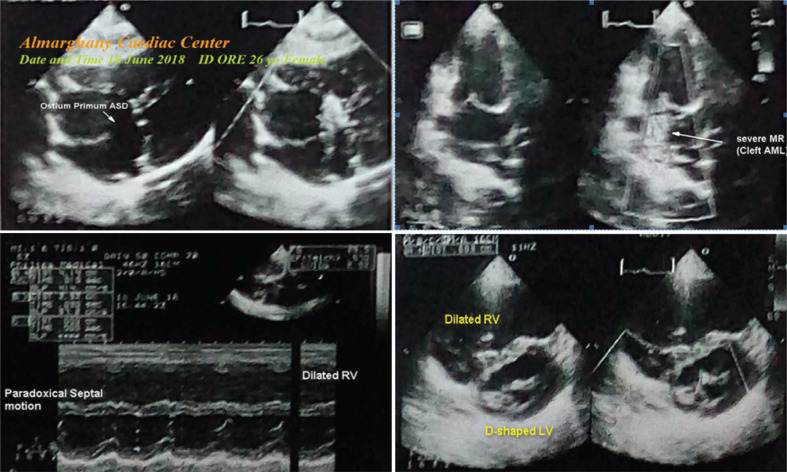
Initial echocardiography was done within 3 days of the patient’s presentation to the outpatient clinic showing an ostium primum atrial septal defect with a left-to-right shunt 2.8 cm in size, severe mitral regurgitation, dilated right ventricle, paradoxical septal motion, tricuspid regurgitation, and mild pulmonary hypertension (47 mmHg) with an ejection fraction of 57%. *Abbreviations:* AML, anterior mitral valve leaflet; ASD, atrial septal defect; LV, left ventricle; MR, mitral regurgitation; RV, right ventricle.
